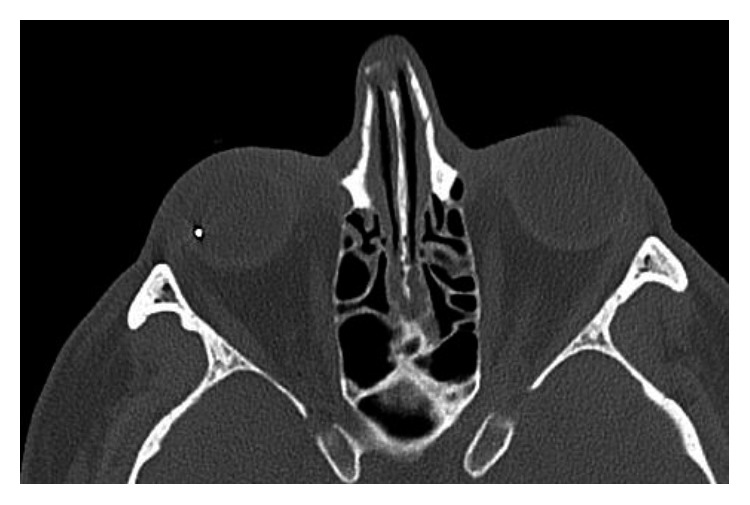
The computed tomography scan of the orbit demonstrates a metallic intraocular foreign body in the patient's right eye.
